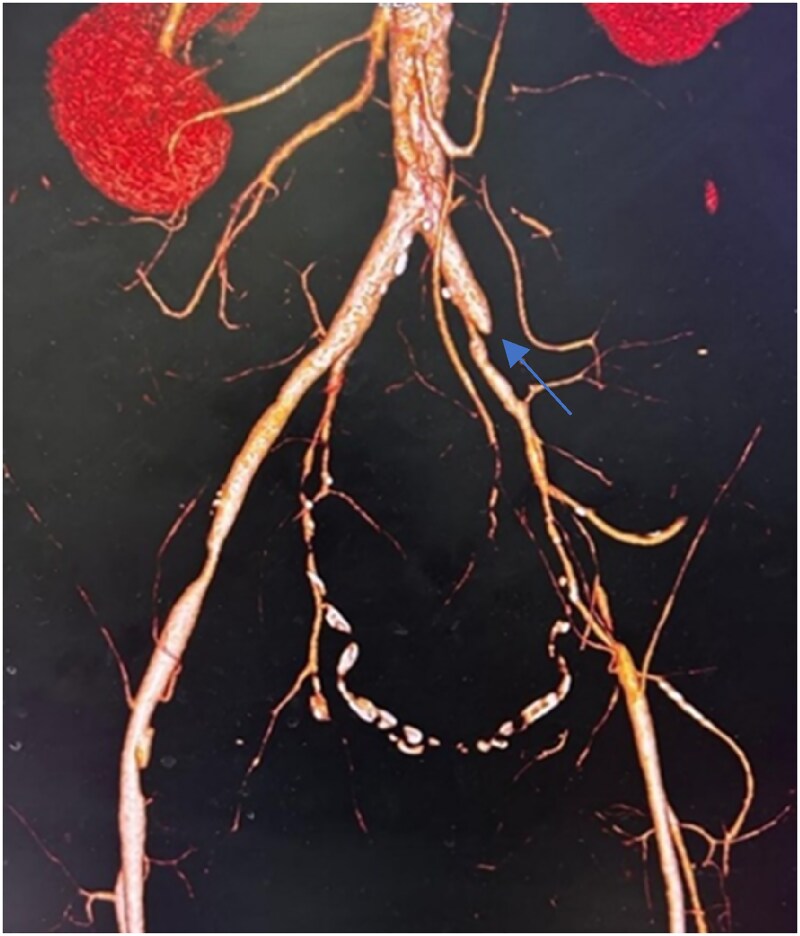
Computed tomography angiography showing complete occlusion of the left external iliac artery (arrow) with 60% stenosis in the right external iliac artery.
